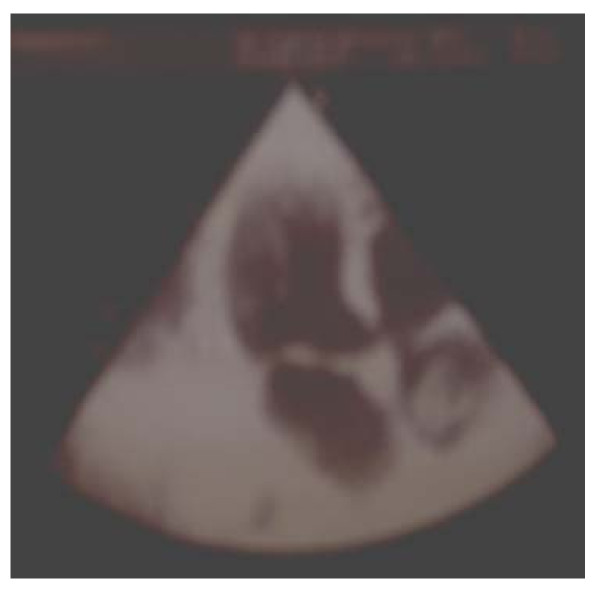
Echocardiography of a right atrial mass, 2 × 2 cm, attached to the atrial septum.
